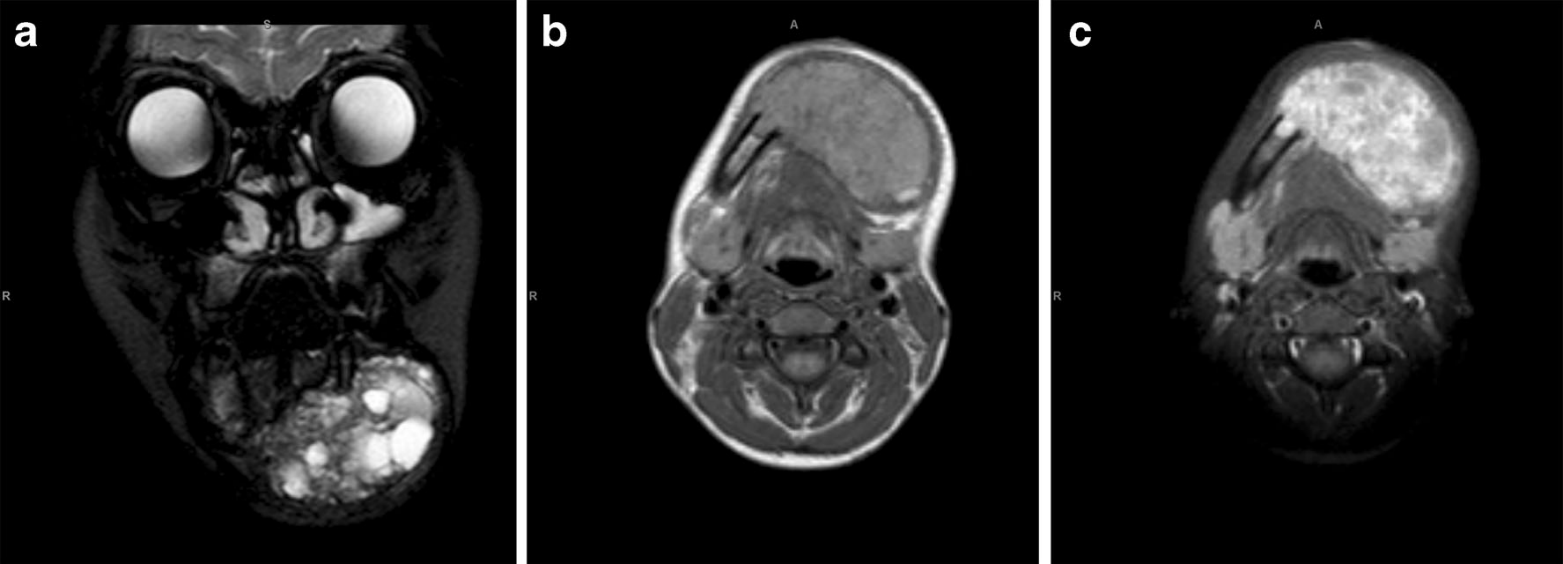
Central giant cell granuloma. Coronal T2-W (a) and axial T1-W (b) MRI show a well-corticated, multi-cystic, expansile mandibular lesion, with solid components which enhance avidly post-contrast (c)
